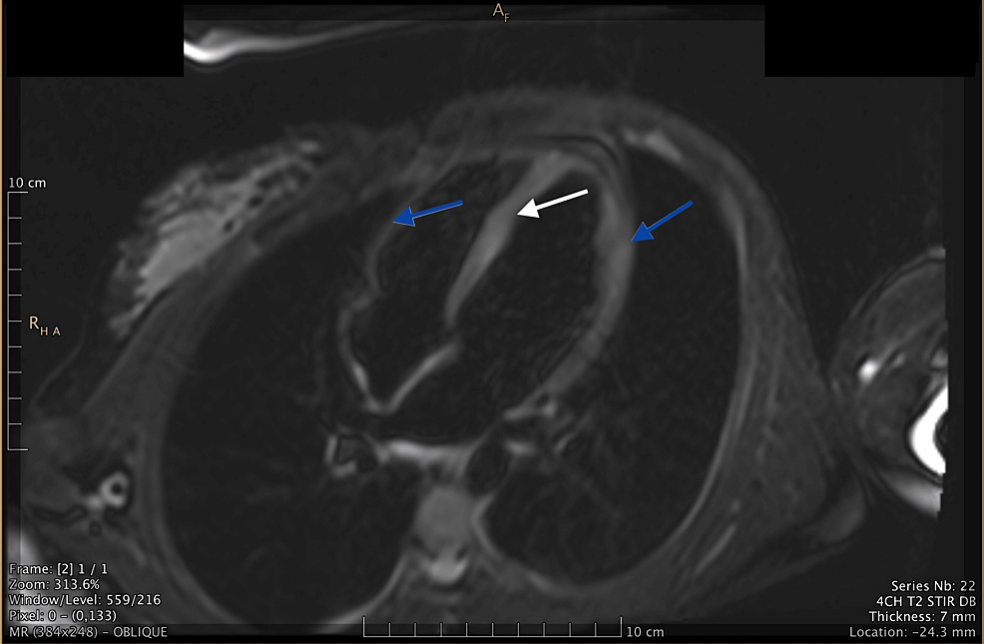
Oblique MRI image demonstrating T2 hyperintense myocardial enhancement of septal and lateral walls characteristic of myocardial edema Blue arrows indicate the areas of T2 hyperintense myocardial enhancement in the lateral walls and white arrow indicates the areas of T2 hyperintense myocardial enhancement in the septum MRI: magnetic resonance imaging
